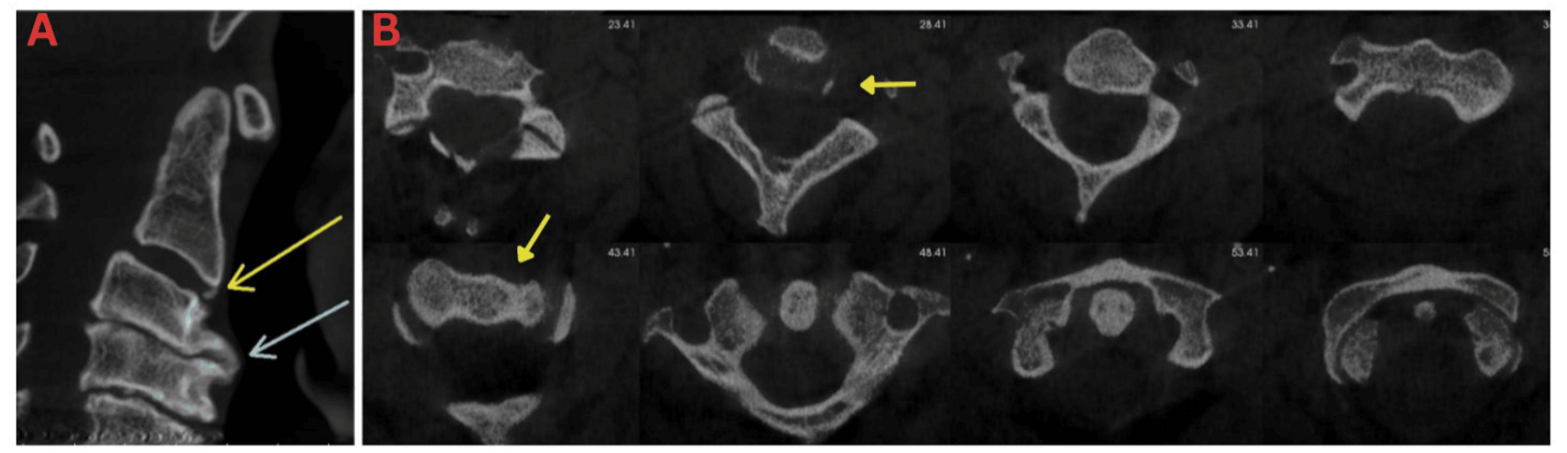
CBCT imaging showing upper cervical vertebrae grade 3. A: Large osteophyte, severe sclerosis of cortical outline, and severe narrowing of intervertebral joint spaces. B: Presence of C1-C2 rotation. CBCT: cone-beam computed tomography.
